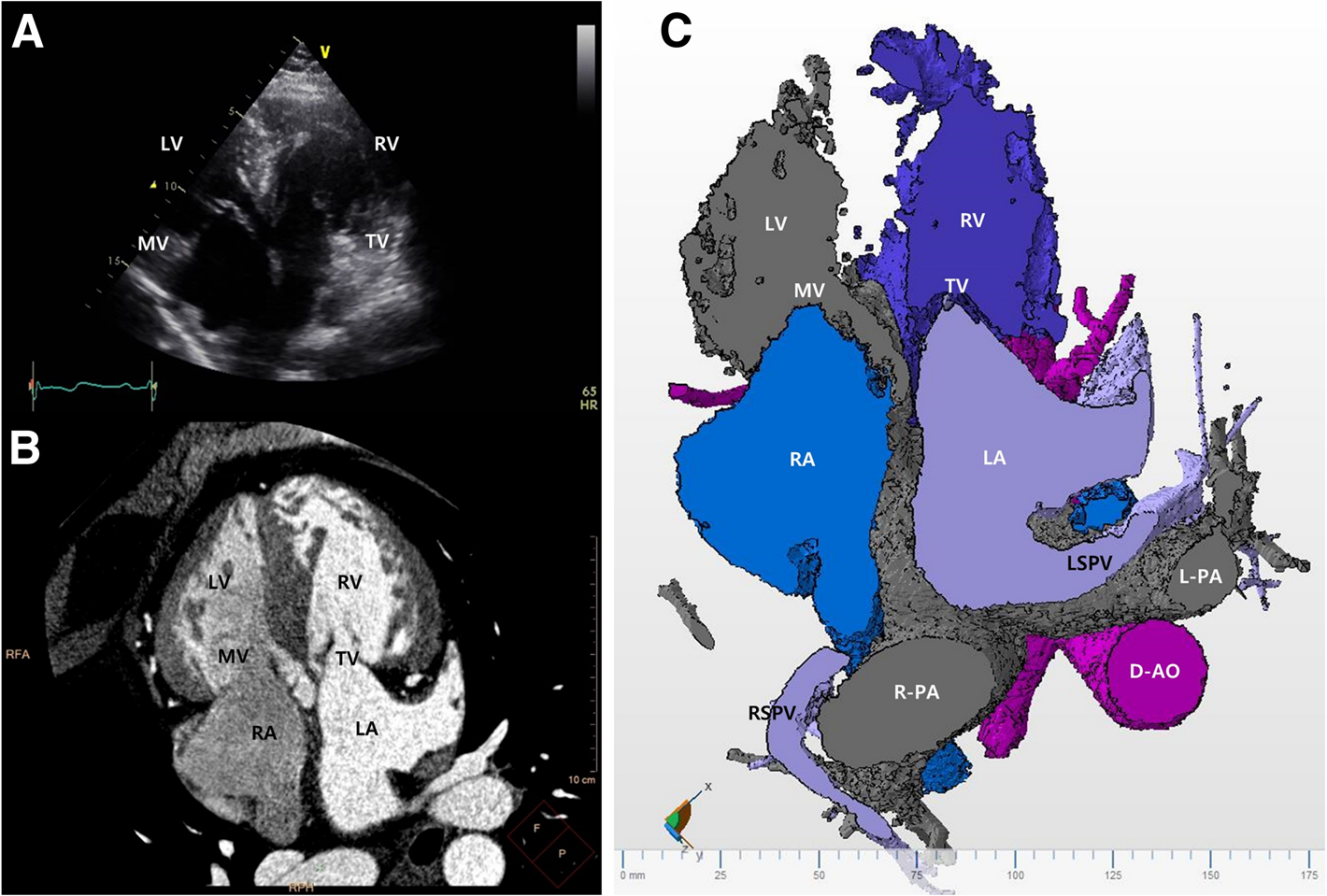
a Transthoracic echocardiography in the four-chamber view plane demonstrates that the left-sided systemic ventricle is the morphologically right ventricle. b Cardiac computed tomography image in a similar plane to that in (a) is shown. The right ventricle has prominently coarse trabeculation and a more apically displaced tricuspid valve than that of the mitral valve. The other smooth-walled right-sided pulmonic ventricle is the morphologically left ventricle. c A three-dimensional model of the cardiac computed tomography at a similar plane to that in (a and b) is shown. *D-AO* descending aorta, *LA* left atrium, *L-PA* left pulmonary artery, *LSPV* left superior pulmonary vein, *LV* left ventricle, *MV* mitral valve, *RA* right atrium, *R-PA* right pulmonary artery, *RSPV* right superior pulmonary vein, *RV* right ventricle, *TV* tricuspid valve
